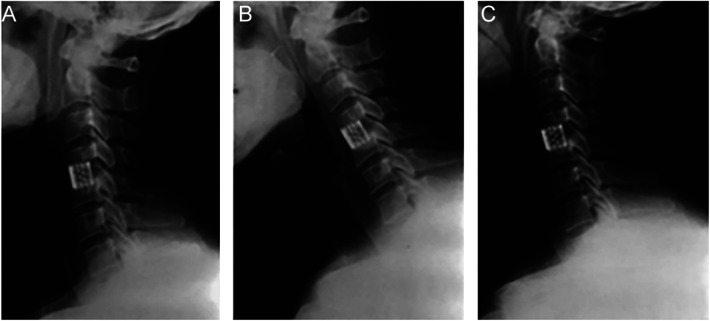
Representative radiographs of a patient undergoing single-level ACDF with a BAK/C cage at C5–6. (A) Preoperative lateral x-ray showing disc space narrowing and osteophyte formation. (B) Lateral x-ray at 1 week post-op showing cage placement and restored intervertebral height. (C) Lateral x-ray at the final 3-year follow-up demonstrating solid bony fusion and maintained alignment.
